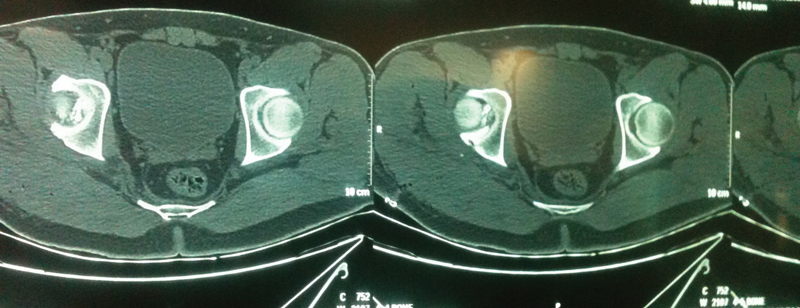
Postreduction axial images from the preoperative computed tomography scan of the affected hip.
